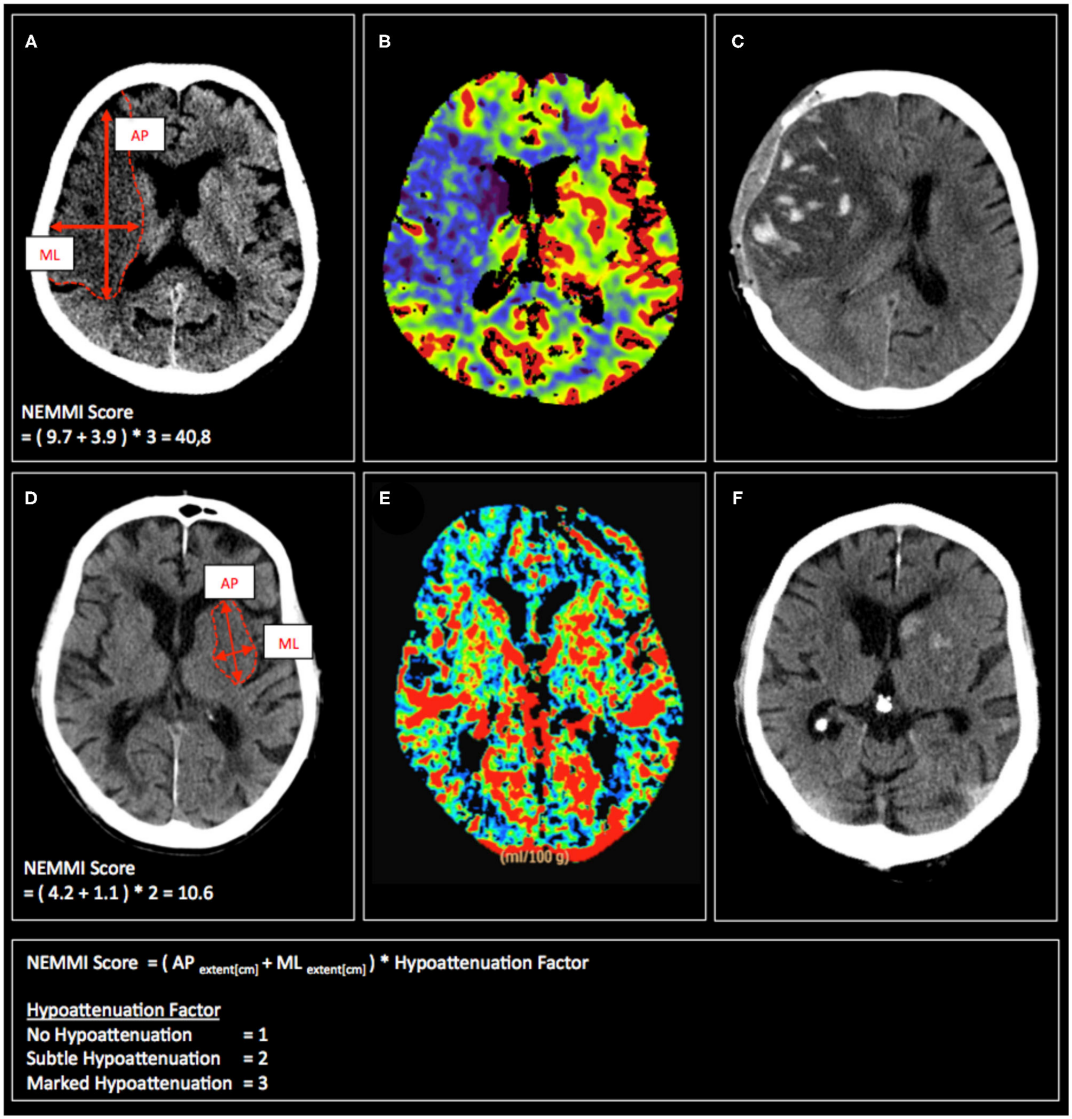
Exemplary admission CT scans (A,D), CBV maps (B,E), and follow-up CT scans (C,F) of patients with acute MCA occlusion, who subsequently develop malignant infarction (upper row) or have a non-malignant clinical course (lower row). Calculation of the respective NEMMI scores as the sum of the maximum AP and ML early lesion diameter multiplicated by the visual hypoattenuation factor is depicted in (A,D).
